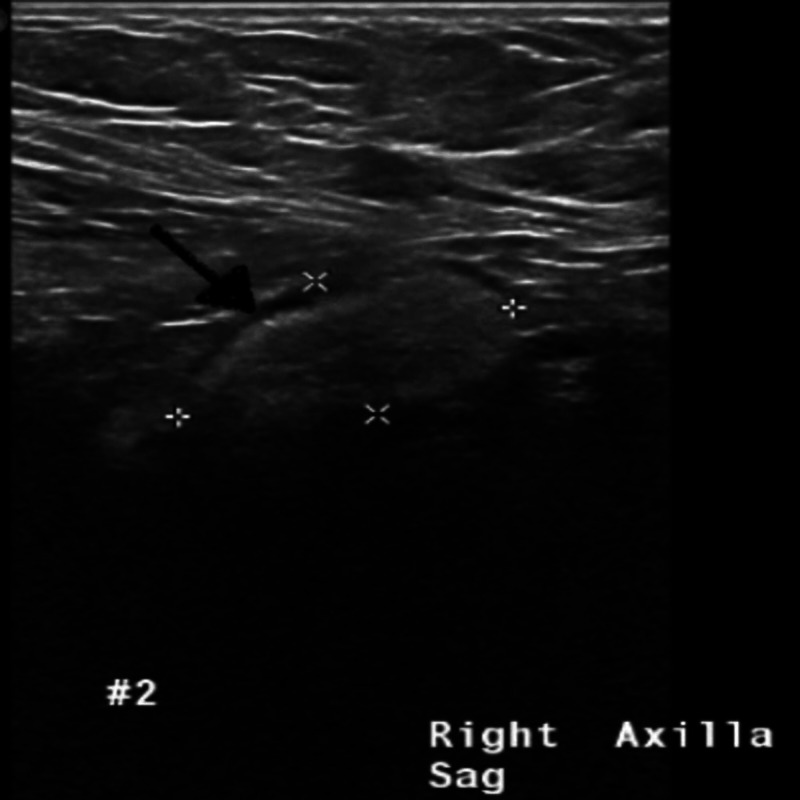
Repeat ultrasound of right axilla showing asymmetric focal density (black arrow pointing the focal density).
